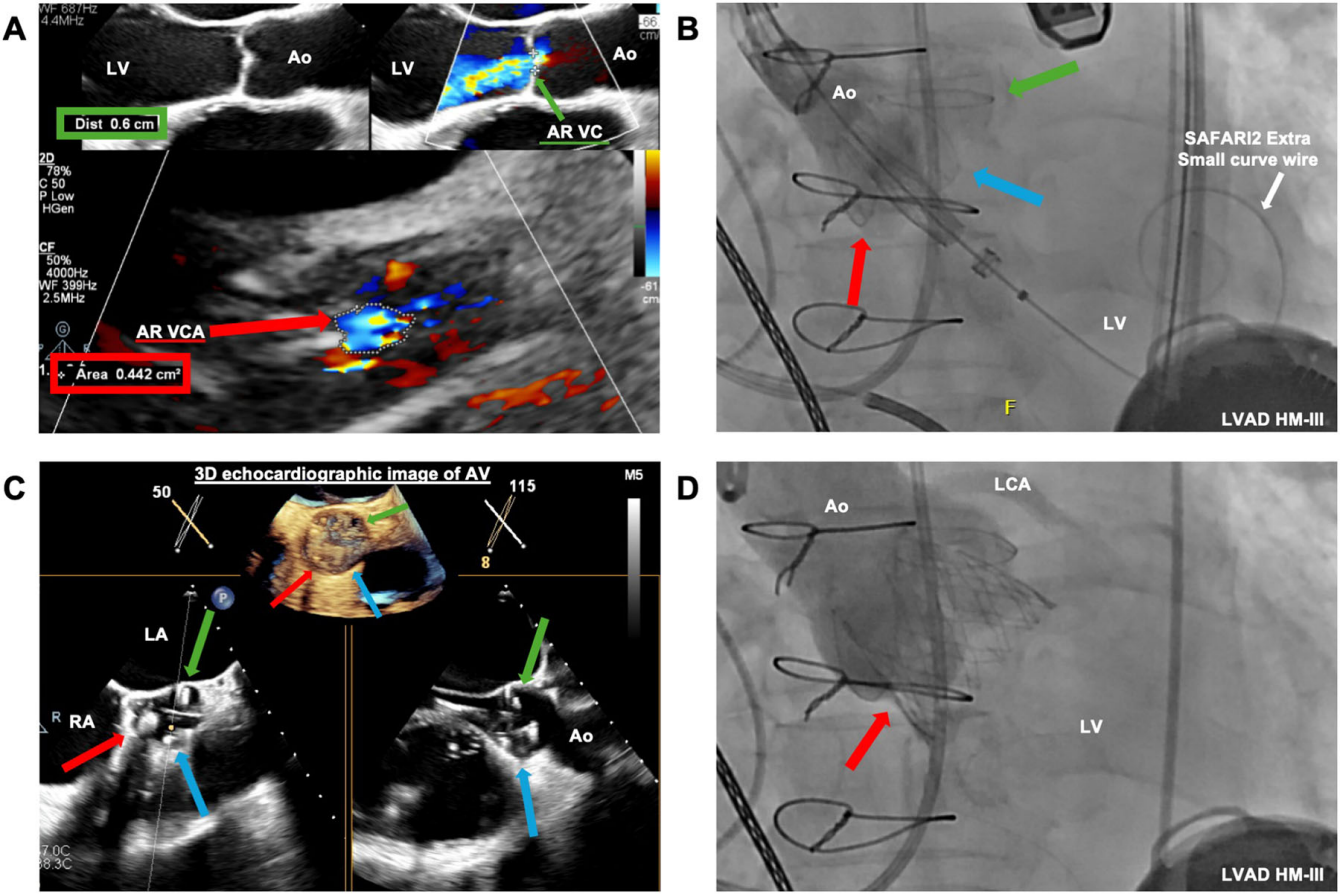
Patient 2. (A) Severe aortic regurgitation with vena contracta (VC) 0.6 cm [red arrow] and VC area (VCA) 0.442 cm2 [green arrow] on transesophageal echocardiography (TEE). (B, C) Claspers of J‐Valve were positioned in aortic sinuses under fluoroscopic and TEE guidance [green arrow—claspers positioned in LCC; blue arrow—claspers positioned in RCC; red arrow—claspers positioned in NCC]. (D) Successful J‐Valve deployment confirmed on fluoroscopy [red arrow—J‐Valve]. [Color figure can be viewed at wileyonlinelibrary.com]
